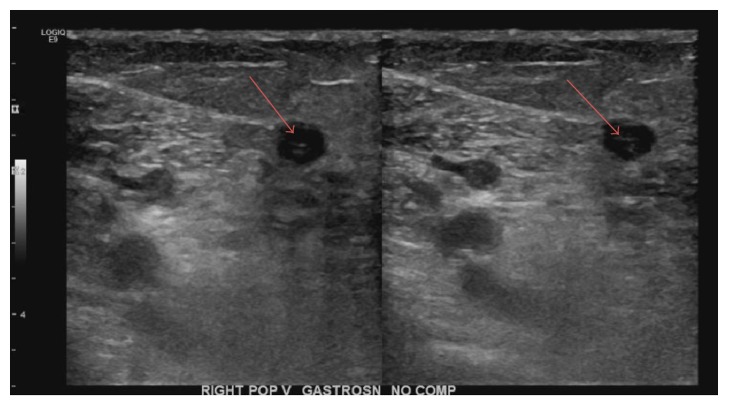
Ultrasound of the right lower extremity showing noncompression of the right popliteal vein as well as a visible thrombus in the lumen (red arrows).
